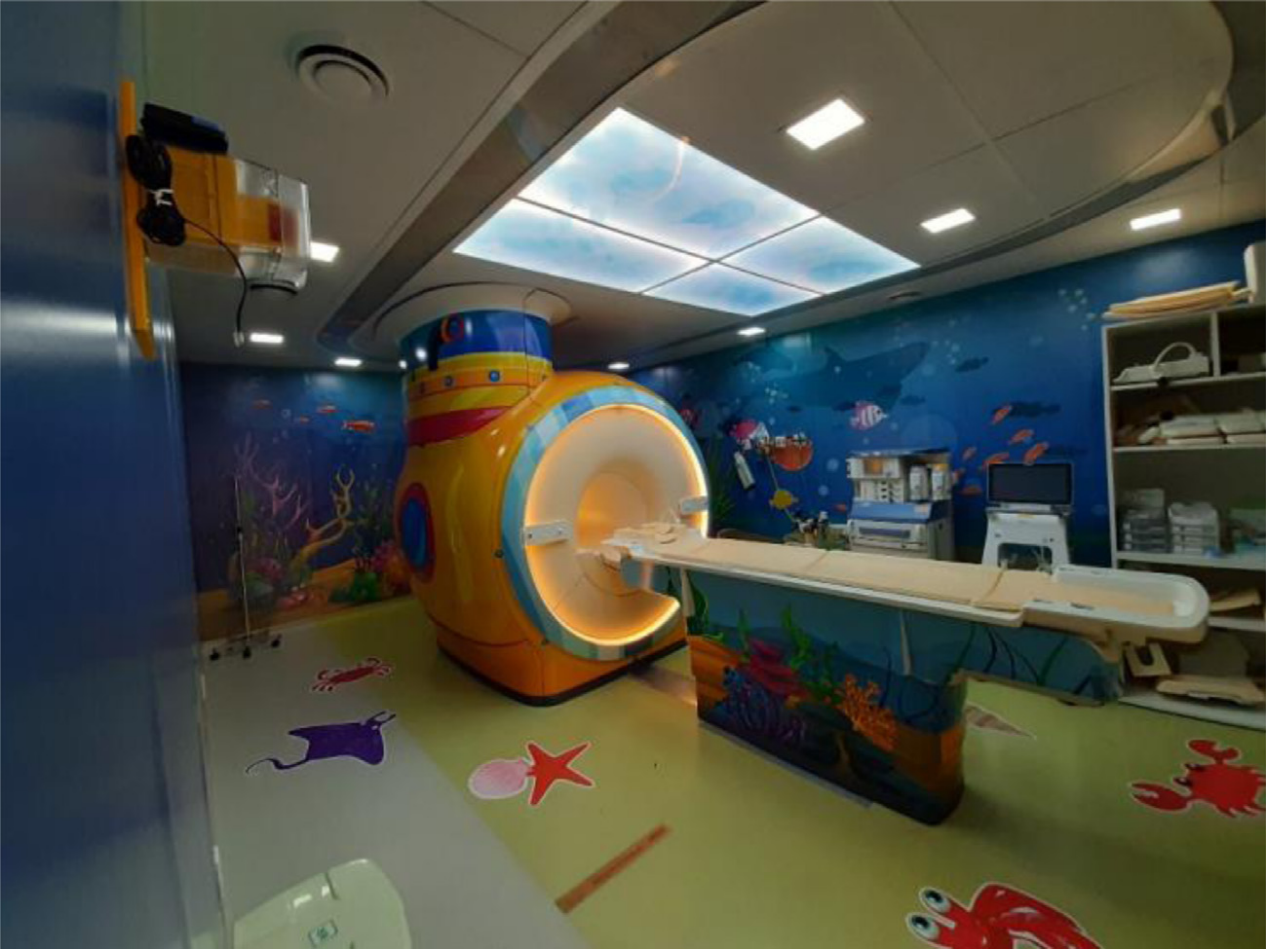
Magnetic Resonance Imaging equipment.
